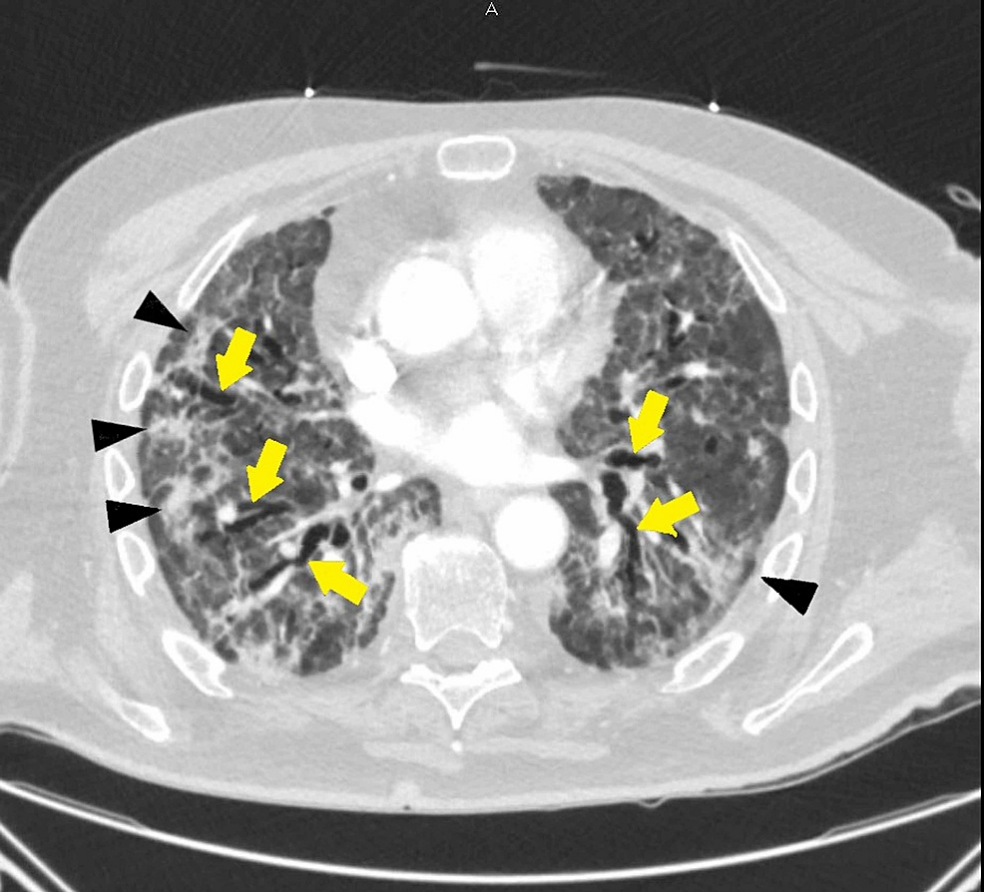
CT of the chest (transverse view) at week six: extensive diffuse bilateral pulmonary ground-glass opacities and air-space disease with architectural distortion bronchiectasis are seen. COVID-19 pneumonia/scarring is also noted. Black arrowheads show ground-glass opacities; yellow arrows show bronchiectasis
